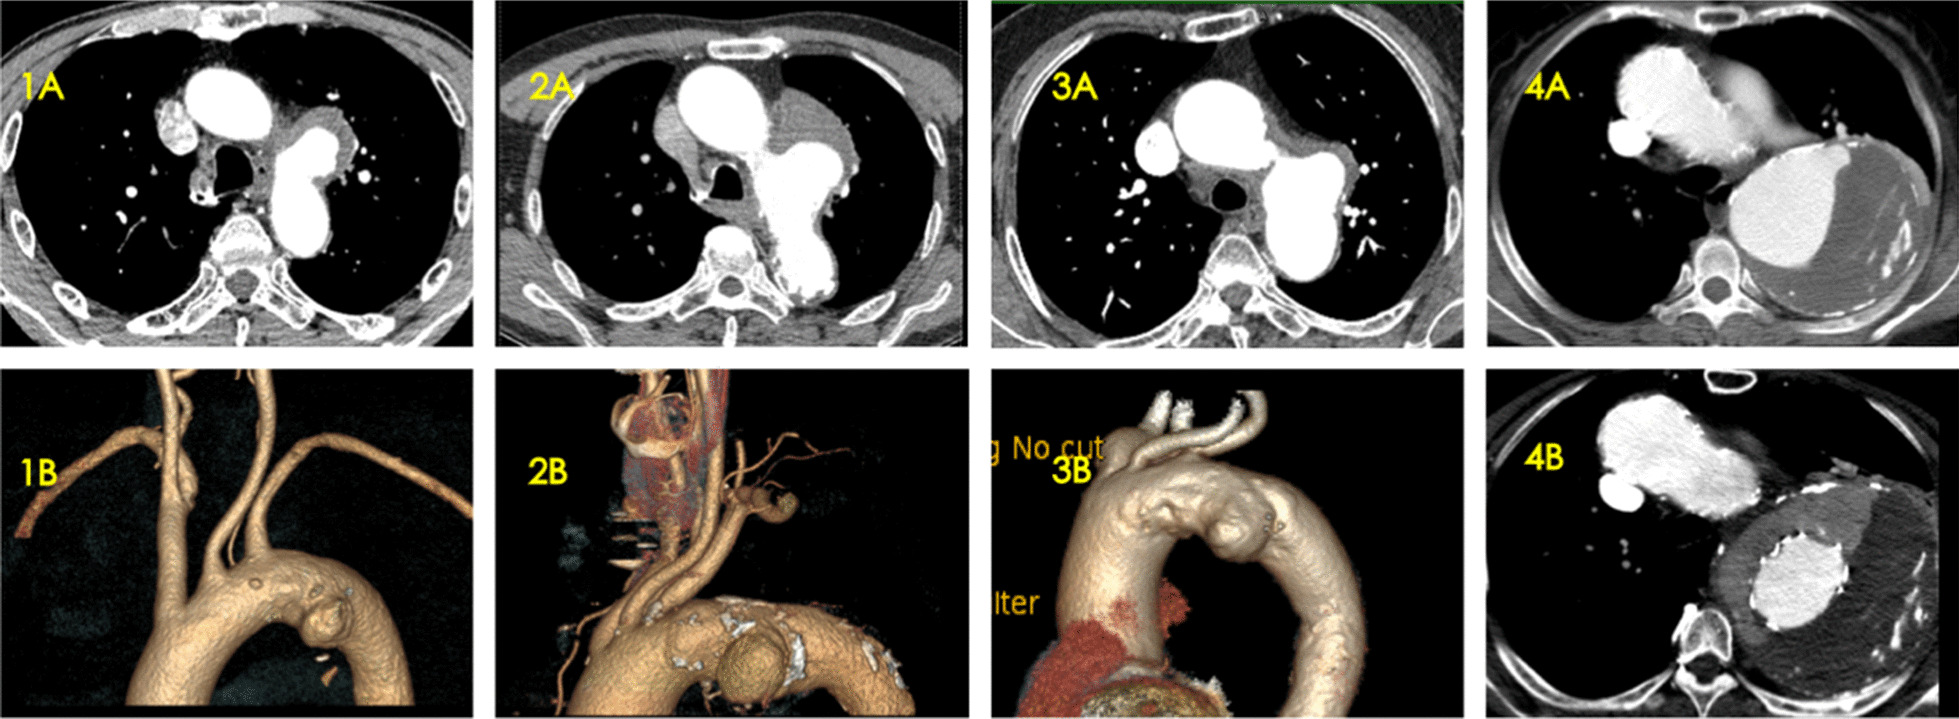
Computed tomography findings. 1A, 2A, 3A: Axial view computed tomography scans of case 1–3; 1B, 2B, 3B: 3D rendering of computed tomography scans of case 1–3; 4A: Axial view computed tomography scans of case 4 before TEVAR (without symptoms of hoarseness); 4B: Axial view computed tomography scans of case 4, 7 months after TEVAR (with symptoms of hoarseness), with type Ia endoleak and increased diameter of the aneurysm. TEVAR, Thoracic endovascular aortic repair
